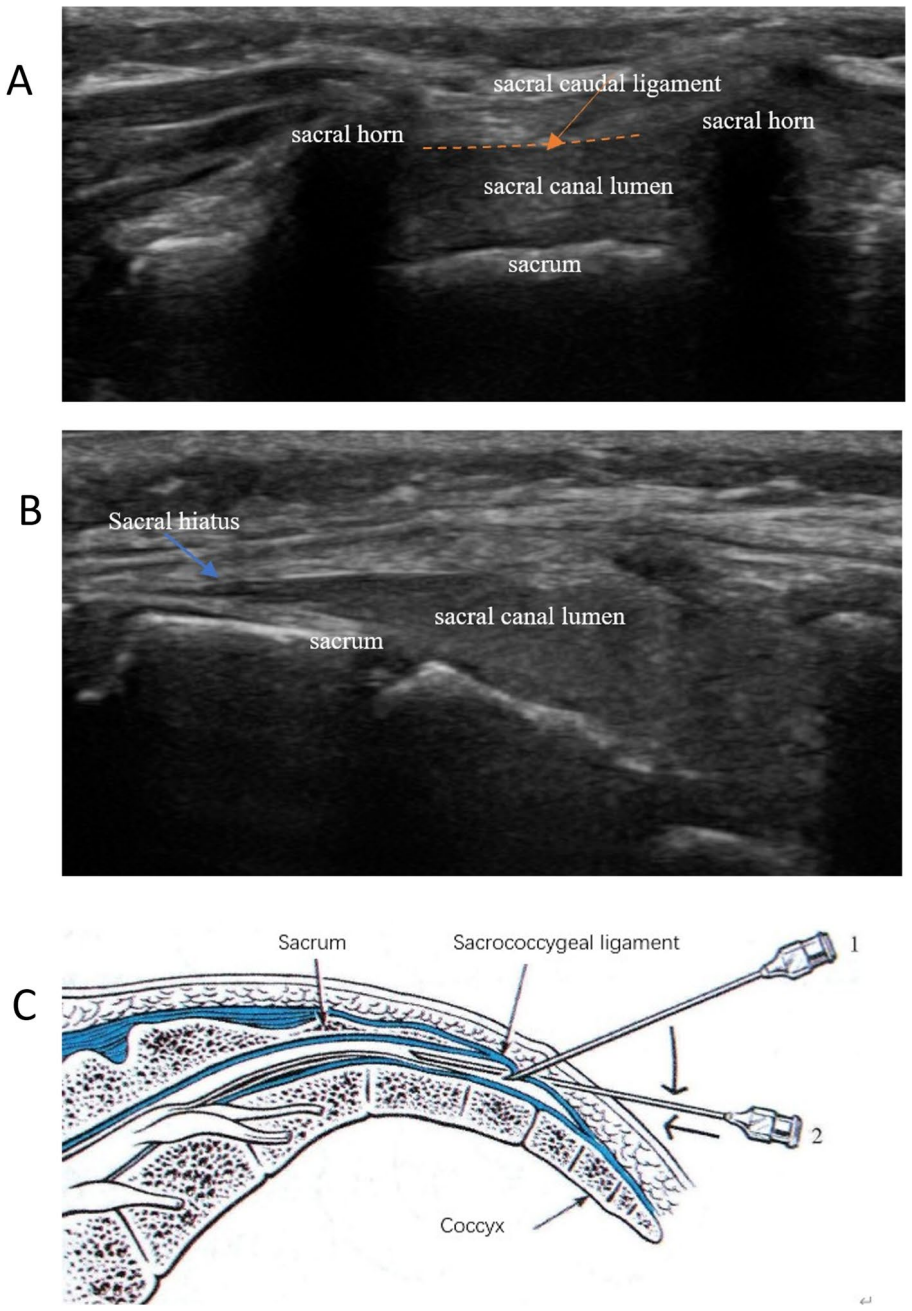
Typical ultrasound images of the sacral canal caudal block anatomy. (A) Short-axis views: frog’s face sign; (B) Long-axis views: staircase sign; (C) Ultrasound-guided intraplanar puncture technique for sacral canal block.
